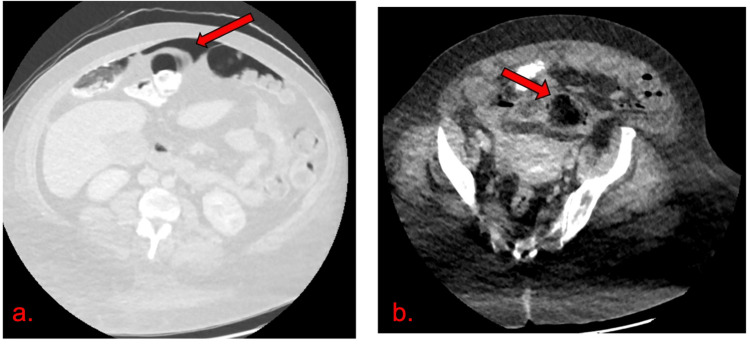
CT angiogram. (a) Evidence of pneumoperitoneum seen within the intra-abdominal cavity using lung window (red arrow). (b) Localized sigmoid inflammation, most likely site of perforation (red arrow).
